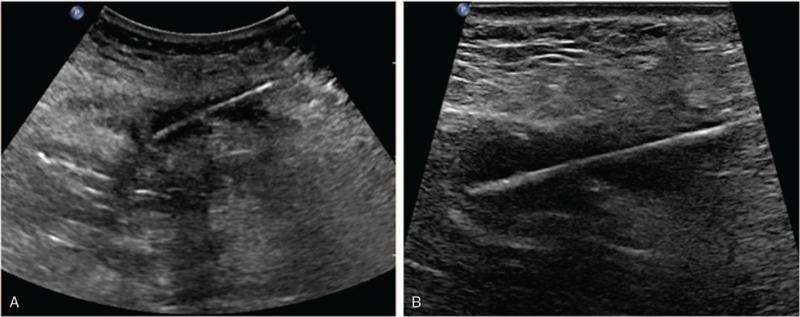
Ultrasound (US) examination of the abdomen. The US examination revealed a 4 cm strip-type hyperechoic object in the muscle of the abdominal wall.
